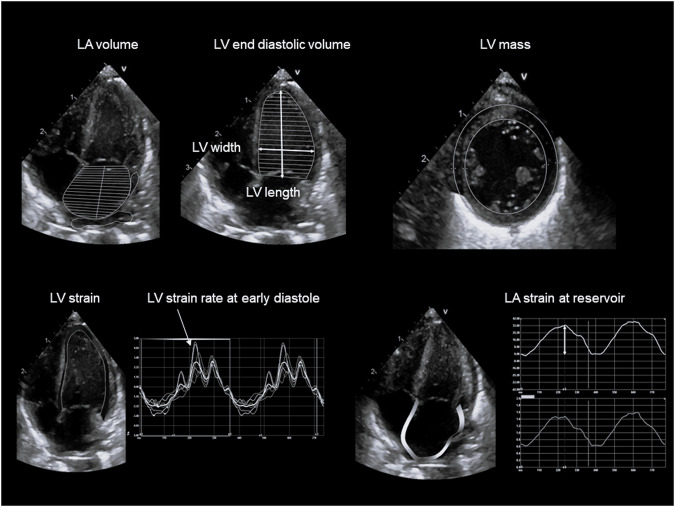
Examples of ultrasound measures of left atrial and ventricular shape and size, mass, longitudinal strain and strain rate.
